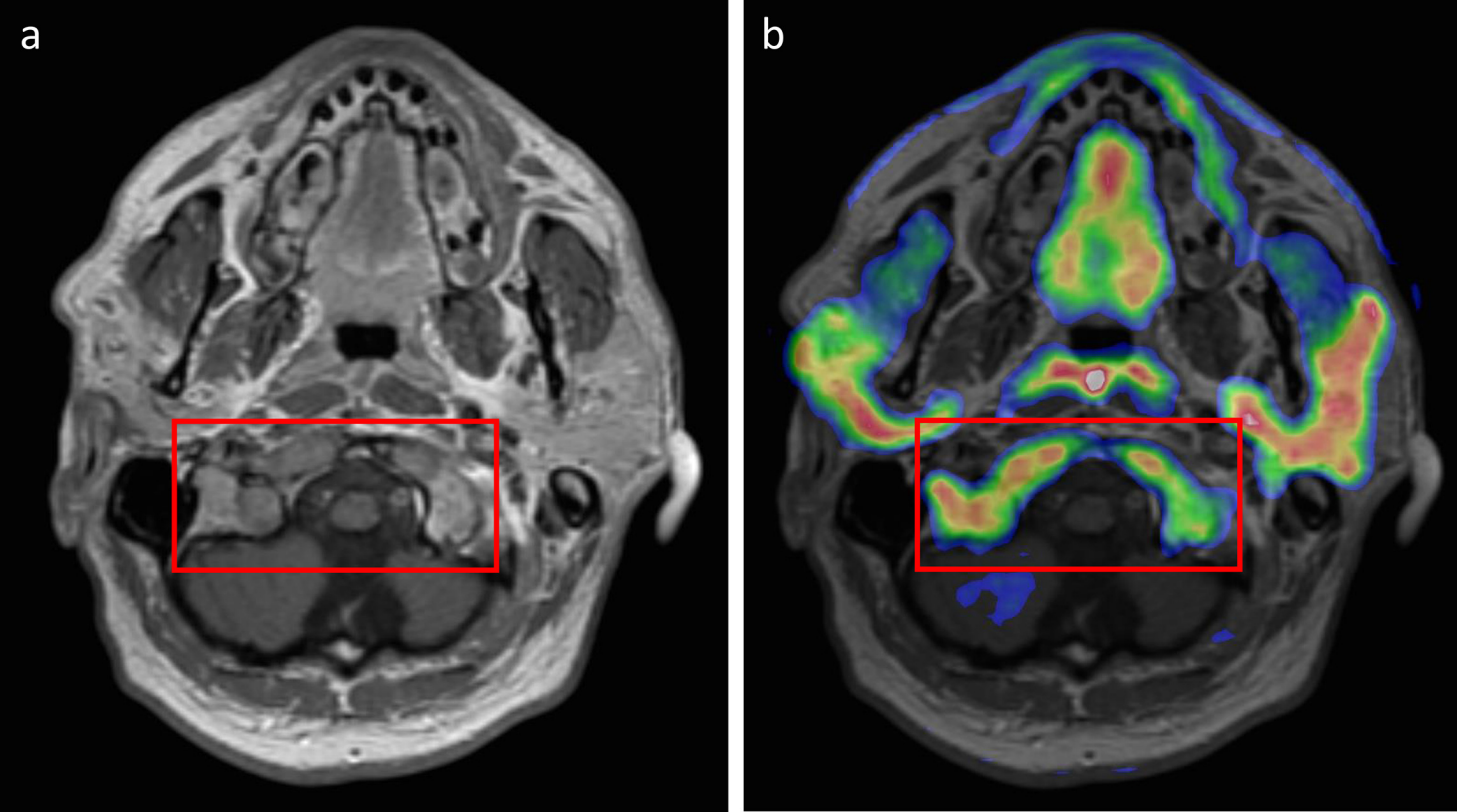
Tumour localisation (indicated by the red box) observed on *T*_1_ weighted axial MRI (a) was confirmed on axial ^11^carbon methionine PET-CT scan (b). Note the normal PET tracer uptake in the parotid glands, seen posterior and lateral to the rami of the mandible, in the posterior wall of the nasopharynx and in the mucosa of the hard palate.
